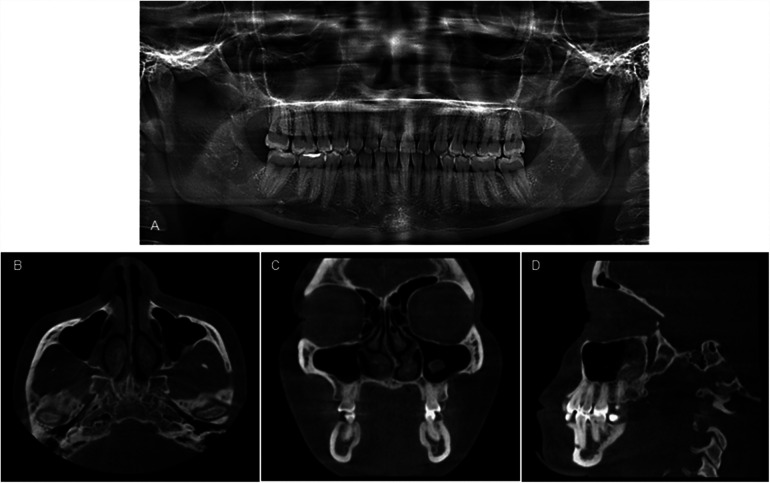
Six-month follow-up imaging. (A) Follow-up orthopantomography showing the left maxillary sinus with no evidence of pathological involvement. Follow-up cone-beam computed tomography (CBCT): (B) axial, (C) coronal, and (D) sagittal views showing a well-defined residual hypodense area compatible with postoperative bone remodeling, with no imaging evidence of recurrence of the dentigerous cyst in the left maxillary sinus.
